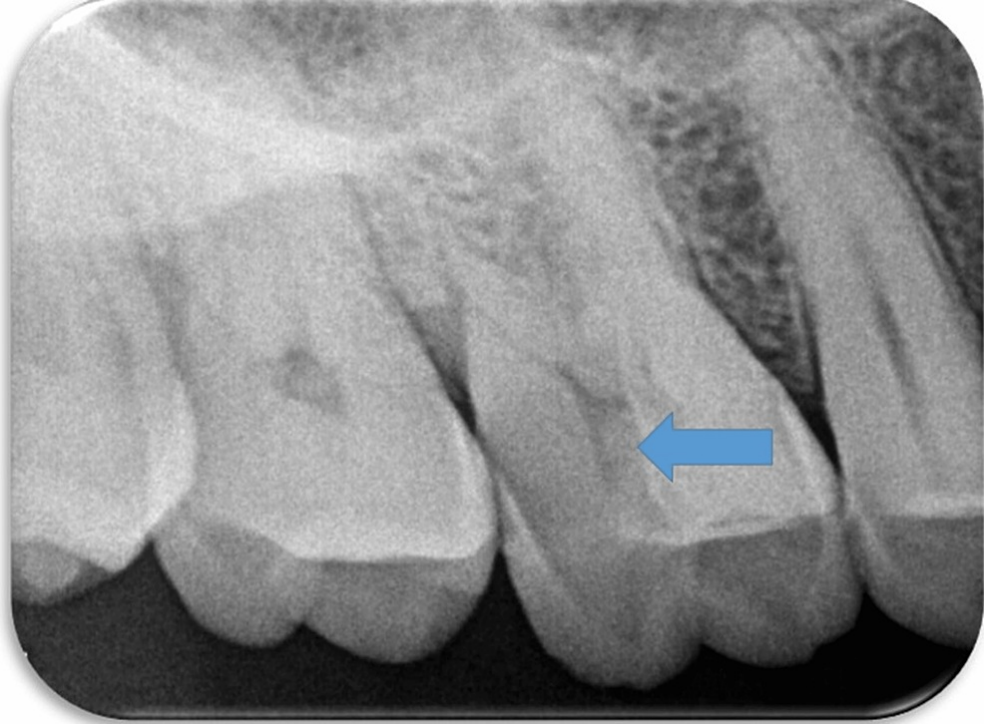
Preoperative radiographic image
